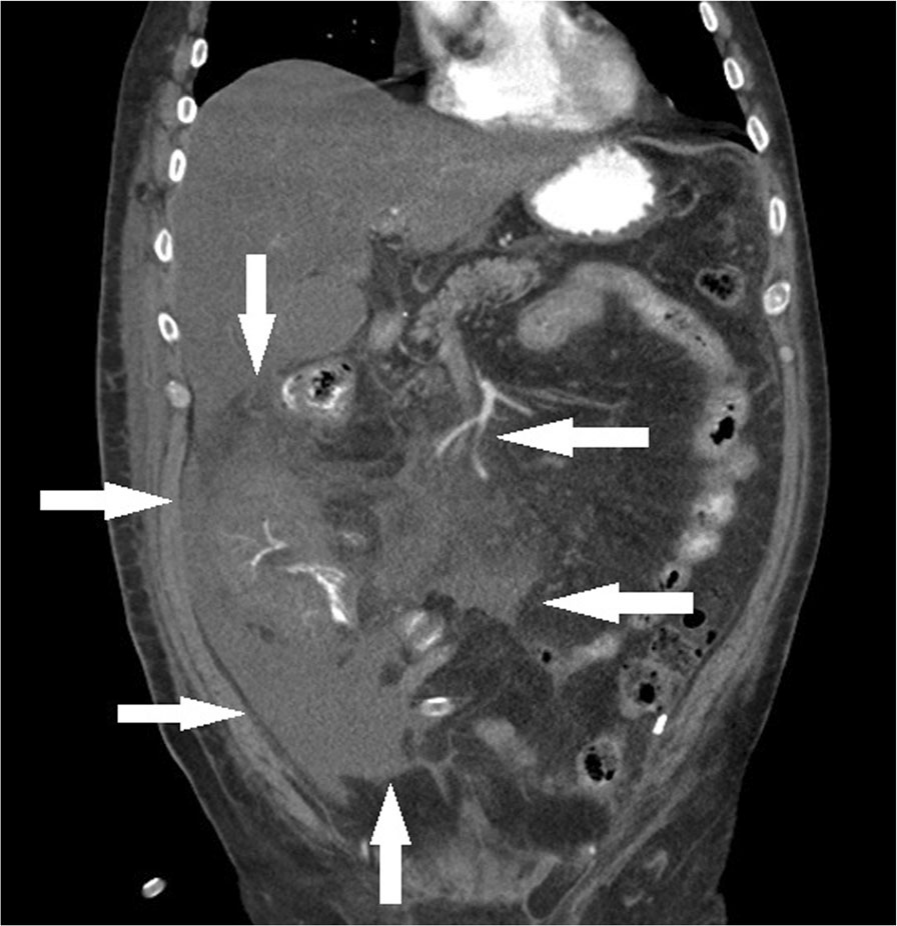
CT scan showing an infiltrate in mesocolon ascendens invading radix mesenterii and the mesenteric vessels
